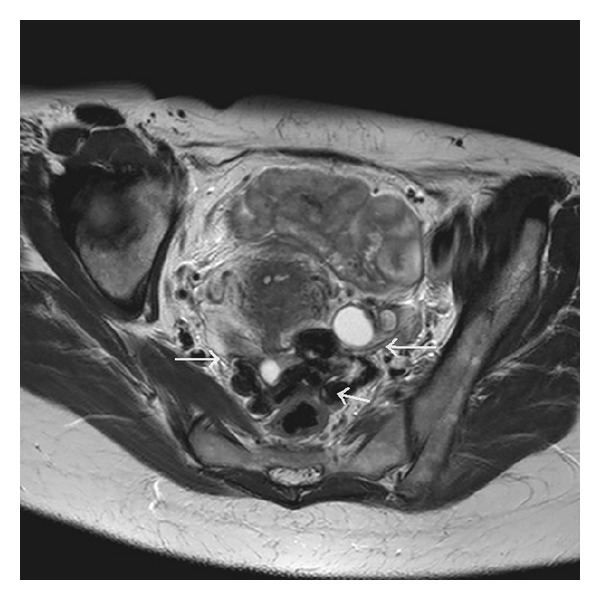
Axial T2-weighted MR image shows polylobular masses in the pouch of Douglas with very low signal intensity.
